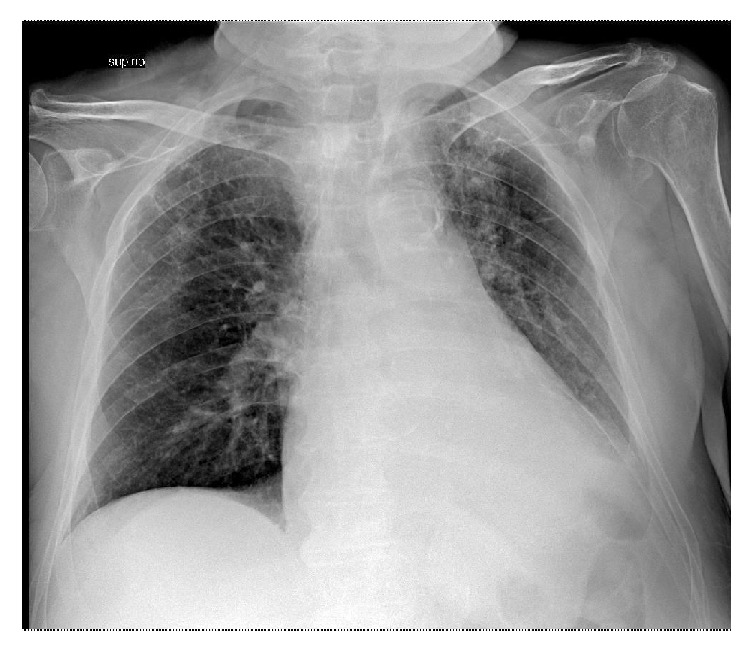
Chest radiography after bronchoscopy.
